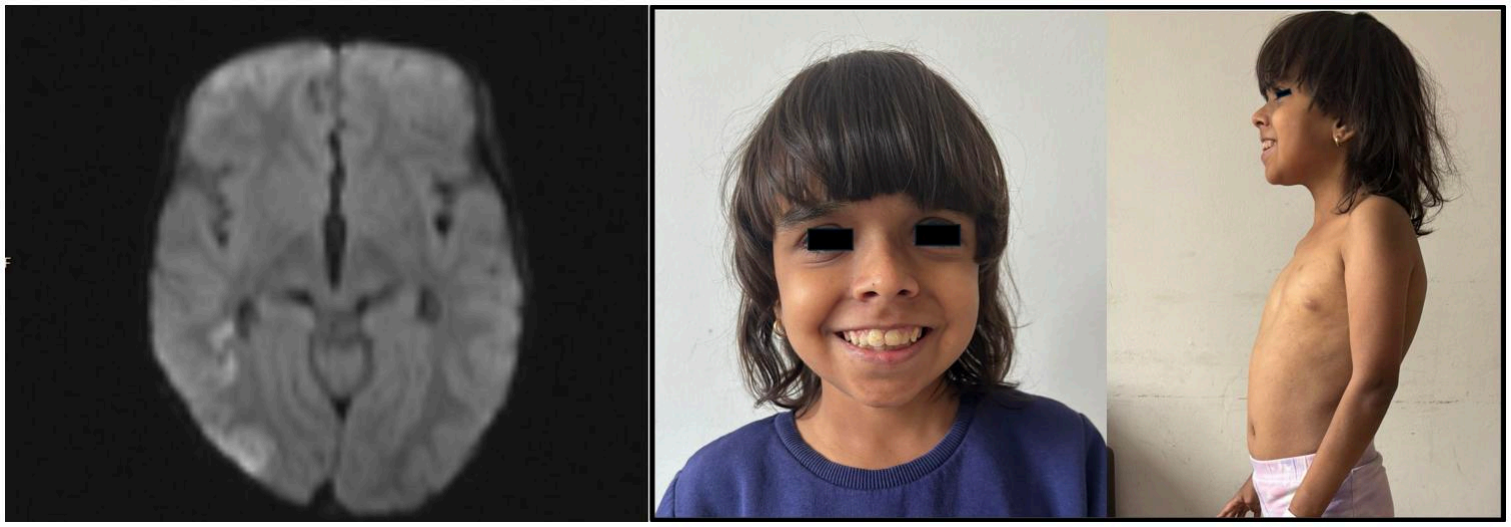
MRI image and dysmorphic features of the patient. Diffusion restriction in the right hemisphere consistent with watershed infarct on MRI and the appearance of the patient at the age of 13 years is remarkable for dysmorphic findings; darkly pigmented skin, coarse hair, rugged facial morphology, hypertelorism, inferiorly positioned palpebral fissures, prominent infraorbital lines, depressed nasal root, low-set ears, micrognathia, pointed chin and pectus excavatum
